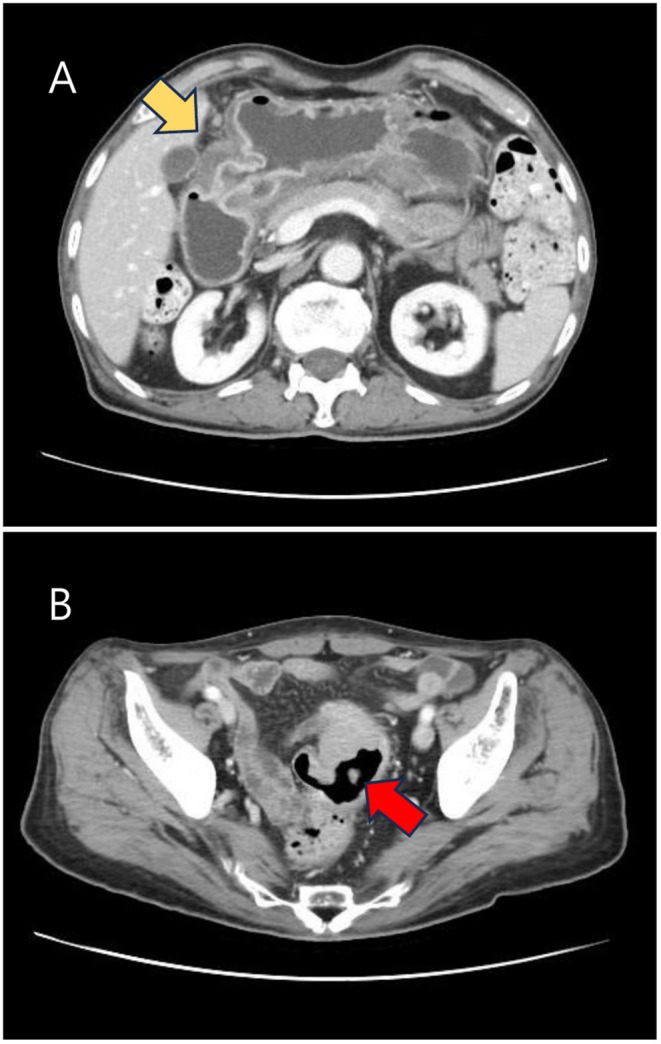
Abdominal computed tomography scan shows stomach cancer involving the antrum to pylorus with peri‐gastric infiltration (yellow arrow) (A) and distal sigmoid colon cancer with peri‐colonic infiltration (red arrow) (B).
